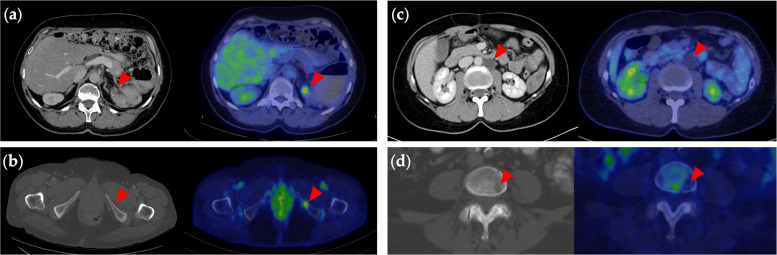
Selected cases demonstrating the added value of PET/CT over conventional imaging in the preoperative evaluation of recurrent ovarian cancer. PET/CT revealed an additional (a) lymph node metastasis (Pt. #7) and (b) a bone metastasis (Pt. #19) not identified on contrast-enhanced CT. Suspicious lesions seen on CT, such as (c) para-aortic cystic lesion (Pt. #21) and (d) lytic vertebral lesion (Pt. #8), were found to be non–FDG-avid on PET/CT, indicating benign etiology
